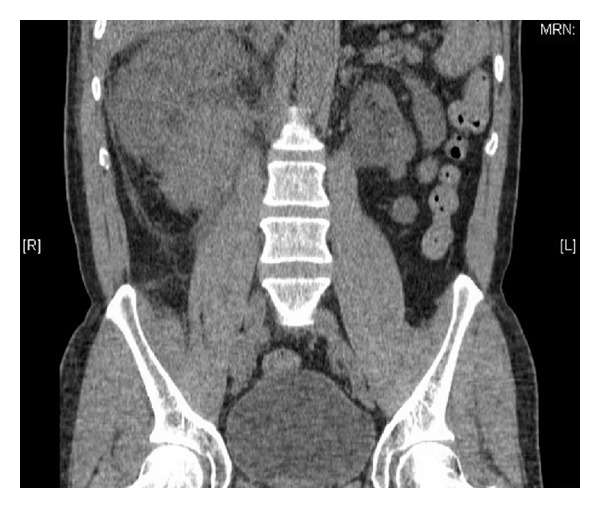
Coronal CT image demonstrating primary plasmacytoma of the kidney.
